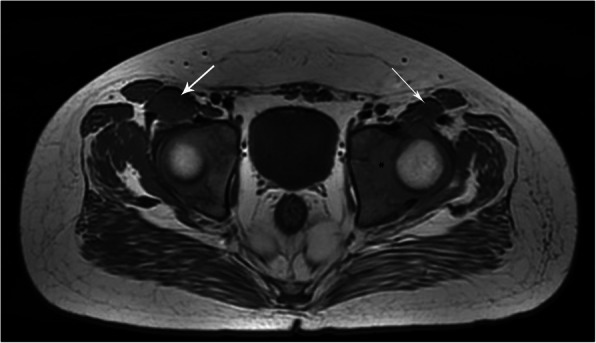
T1WI: Case of a 7-month-old girl with atrophy of the left iliopsoas muscle (*arrow*) and the right normal muscle (*arrow*) shows an intermediate-intensity signal on T1-weighted axial section images. The left iliopsoas muscle width decreases
